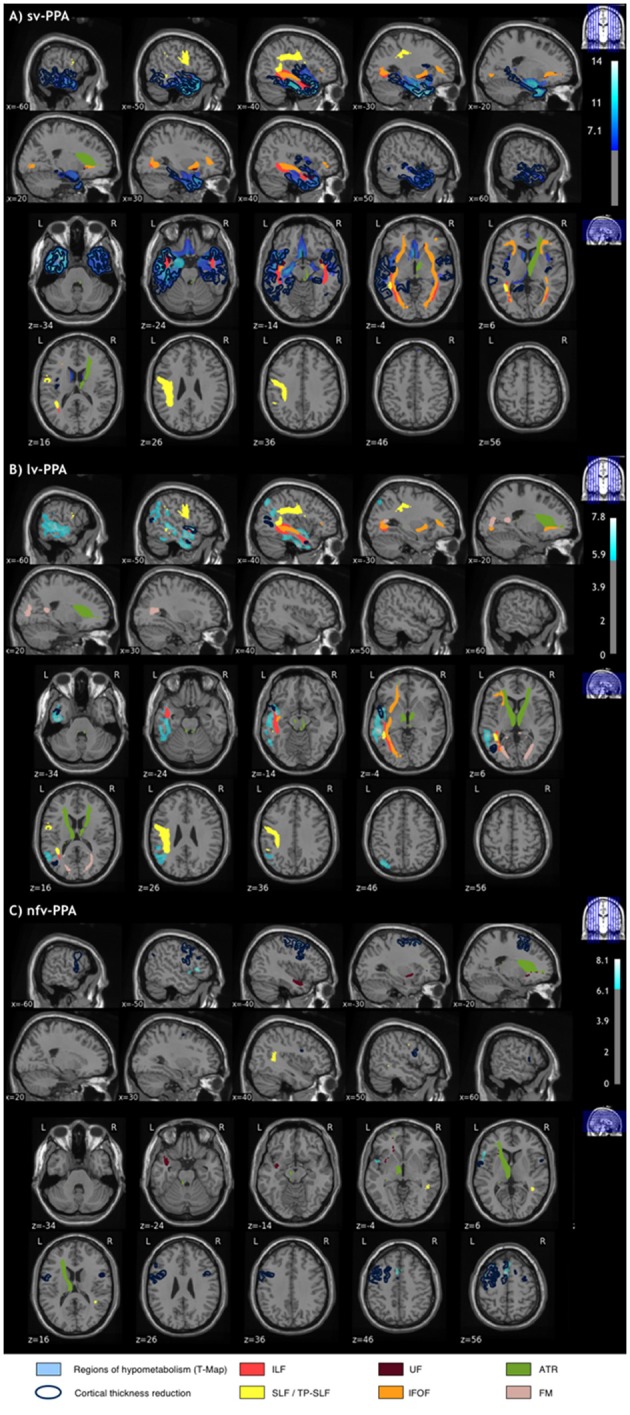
Alterations across the three imaging modalities (T1-cortical thickness, FDG-PET, DWI-tracts) in (A) sv-PPA (B) lv-PPA (C) nfv-PPA patients, compared to healthy controls. Significantly altered areas correspond to a corrected *p*-value of 0.05 for all modalities. The correction for multiple comparisons used FWE at vertex-level for T1, FWE at peak-level for PET, and Bonferroni for DWI.
